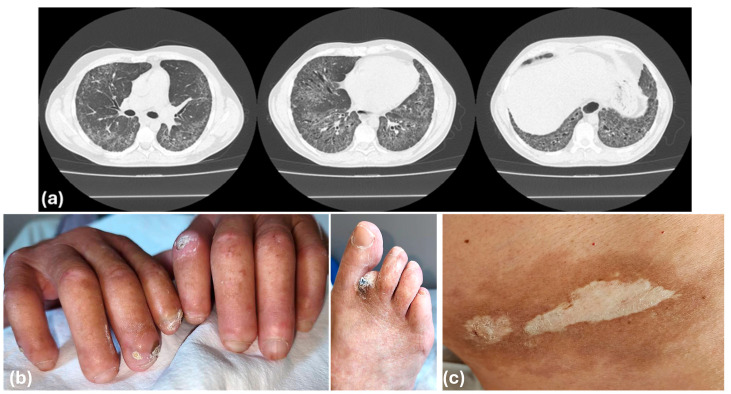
(a) High-resolution computed tomography (HRCT) scan of the chest in a patient with systemic sclerosis-associated interstitial lung disease (SSc-ILD). The scan demonstrates imaging features consistent with a fibrotic non-specific interstitial pneumonia (NSIP) pattern, including bilateral, basal-predominant ground-glass opacities with relative subpleural sparing, reticulation, and traction bronchiectasis. Additionally, a dilated esophagus is visible, a common extrathoracic manifestation of systemic sclerosis. (b) Scleroderma in the fingers, toes, and (c) in the abdominal area with characteristic central fibrosis. (Courtesy of the archive of Prof. Argyris Tzouvelekis and Dr. Katerina Grafanaki).
